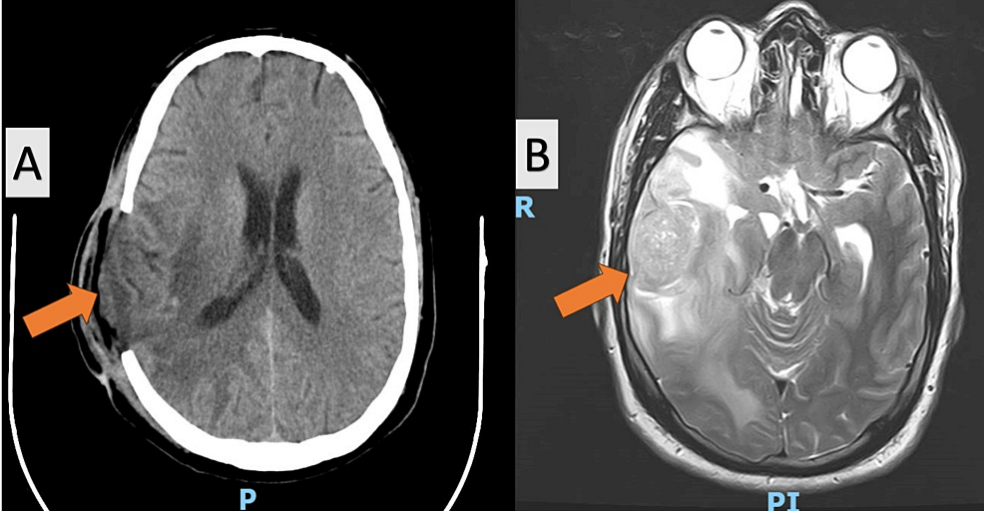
(A) Postoperative CT scan that shows no active hemorrhage, (B) preoperative MRI scan
